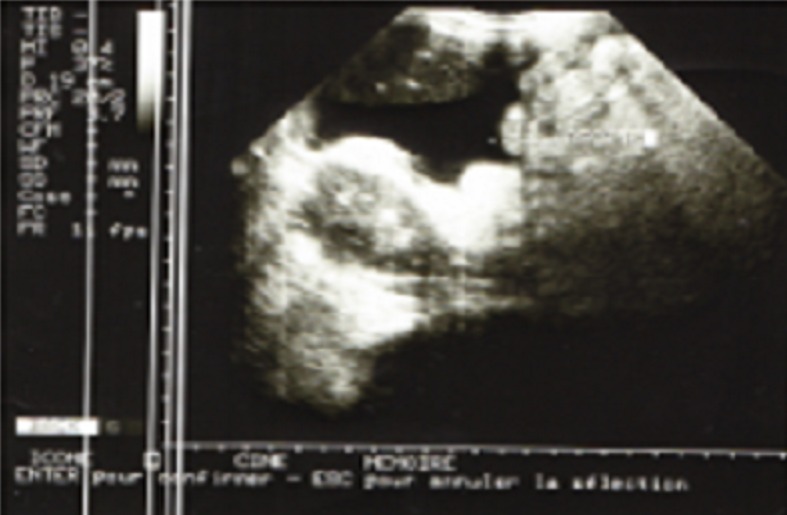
Obstetric ultrasound image showing a pregnancy at 23 weeks of amenorrhoea with ascites filling the interhepato-diaphragmatic space
